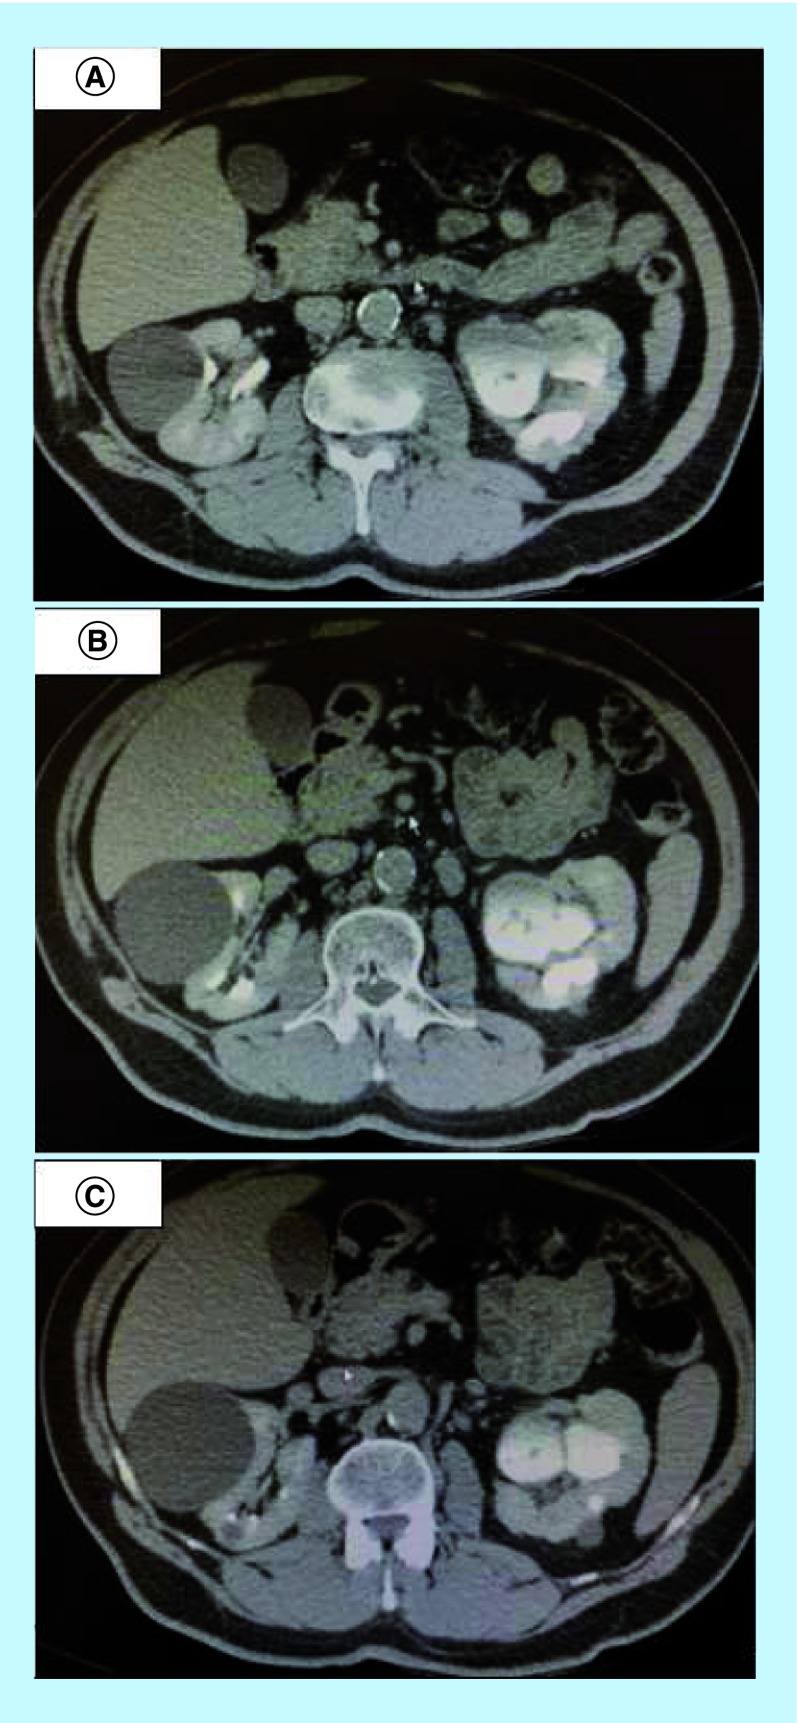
(A–C) Abdominal computed tomography scan showing multiple nonenhancing filling defects on the left renal pelvis at delayed phase before treatment with teicoplanin.
